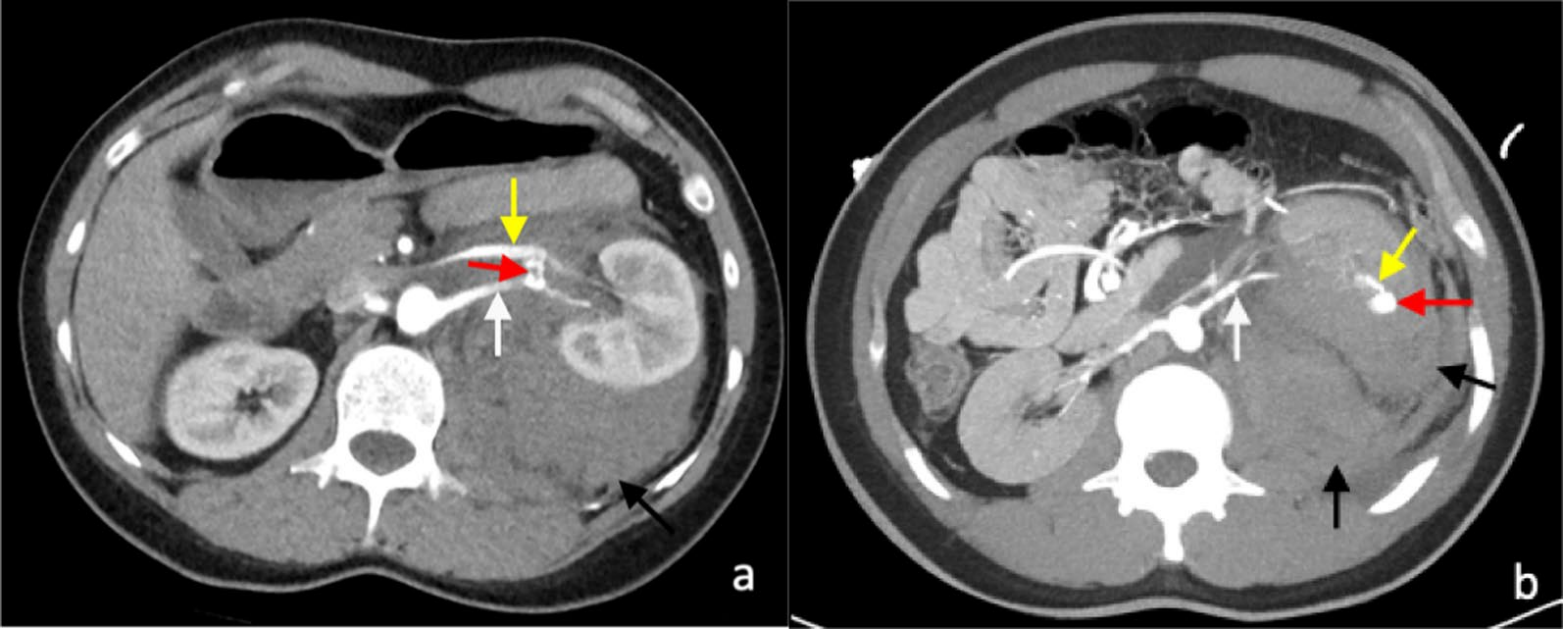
Post-contrast arterial phase axial CT images of vascular complications following non-targeted ultrasound-guided 14-gauge core biopsies of the left kidney in two patients. a Arteriovenous fistula. There is a fistulous connection (red arrow) between the left renal artery (white arrow) and the left renal vein (yellow) with associated early arterialised enhancement of the proximal left renal vein, medial to the arteriovenous fistula. There is an associated retroperitoneal haematoma (black arrow). b Arterial pseudoaneurysm. A small pseudoaneurysm (red arrow) arising from a segmental branch of the left renal artery (yellow arrow) is visualised as an adjacent small focus of rounded arterial enhancement (red arrow). The main left renal artery is also visible (white arrow). There is an associated left perinephric and retroperitoneal haematoma (black arrows)
